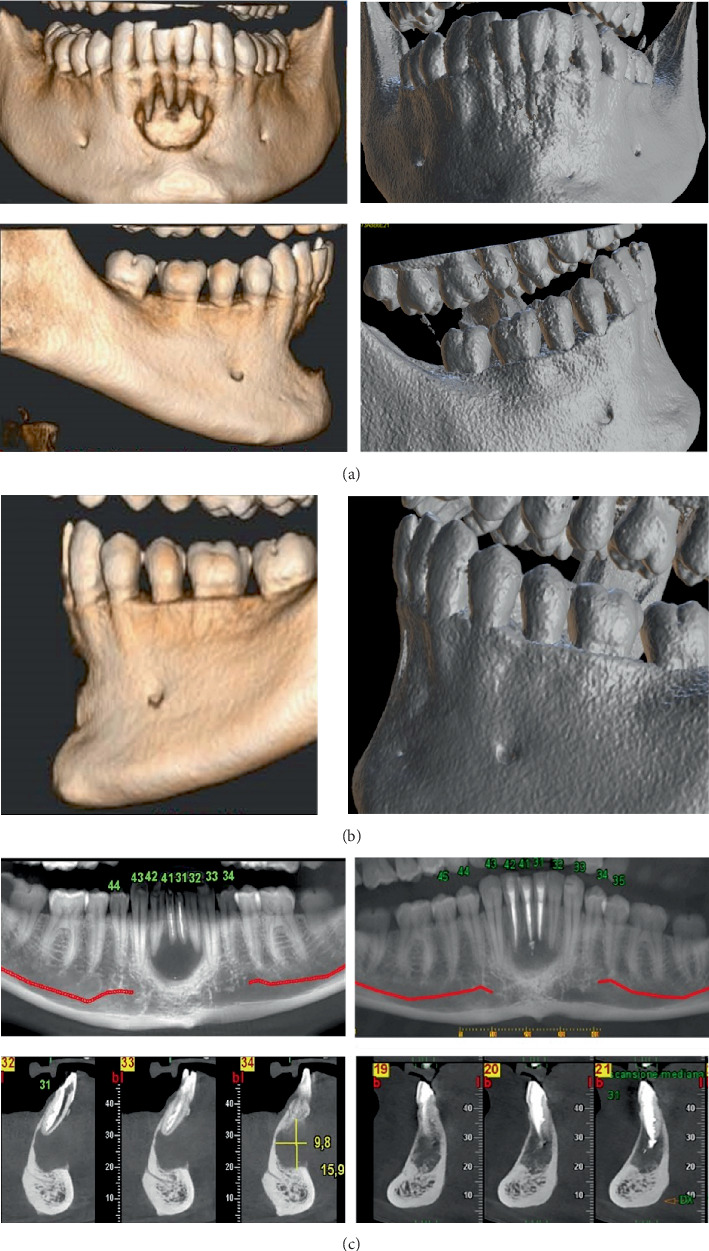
Preoperative and 6-month posttreatment CBCT analysis. (a) 3D reconstruction of the preoperative CBCT scan showing the large periapical lesion and cortical bone destruction and the 3D reconstruction at 6 months posttreatment demonstrating substantial buccal bone regeneration. (b) Axial sections of the CBCT scans pretreatment and posttreatment, highlighting the reduction in lesion size and the reformation of an initial bone structure. (c) Sagittal sections of the CBCT scans pretreatment and posttreatment providing a detailed view of the vestibular cortical bone regeneration.
